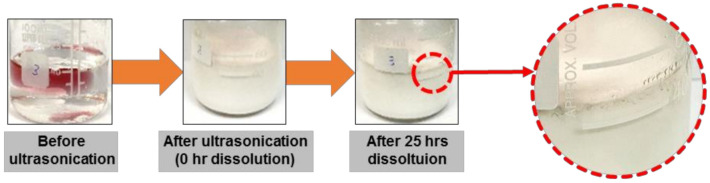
Photographs showing the formation change of the P-BED composed of PFC, anionic surfactant, 0.37 M H_2_SO_4_, and 0.13 mM KMnO_4_ before ultrasonication and during the dissolution test.
